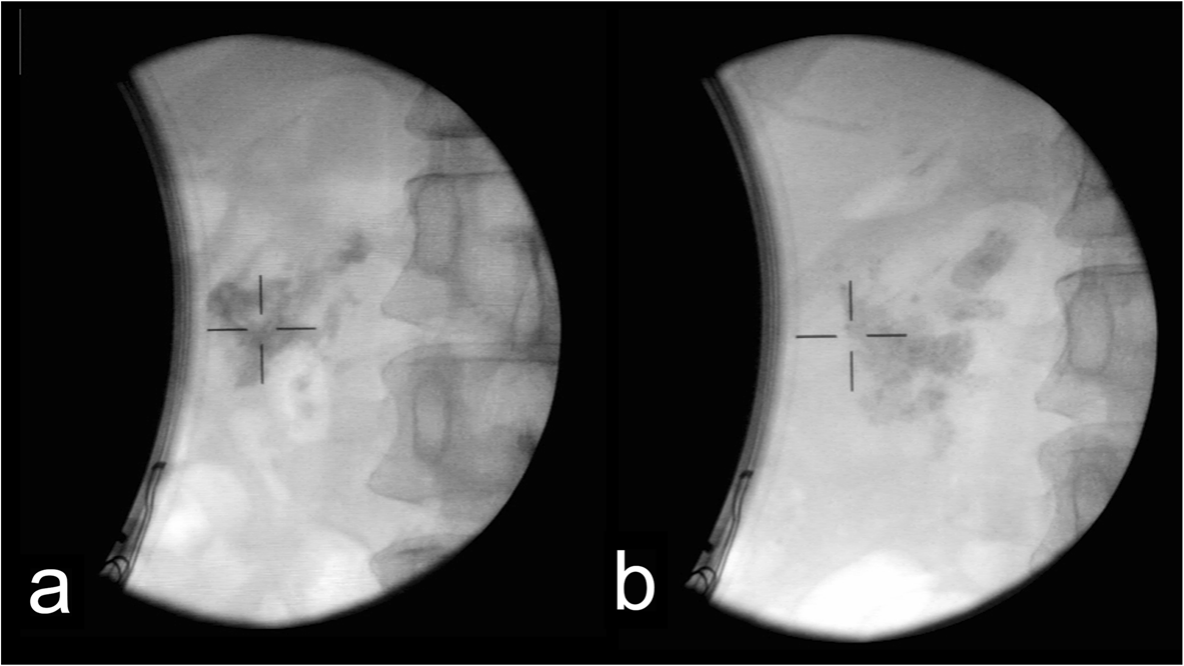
X-ray image of the extracorporeal shock wave lithotripsy was photographed in a 30°-right supine position of patients. a The X-ray image for pancreatic stone of the patient before the first session of P-ESWL. b The X-ray image for pancreatic stone of the patient after the last session of P-ESWL
